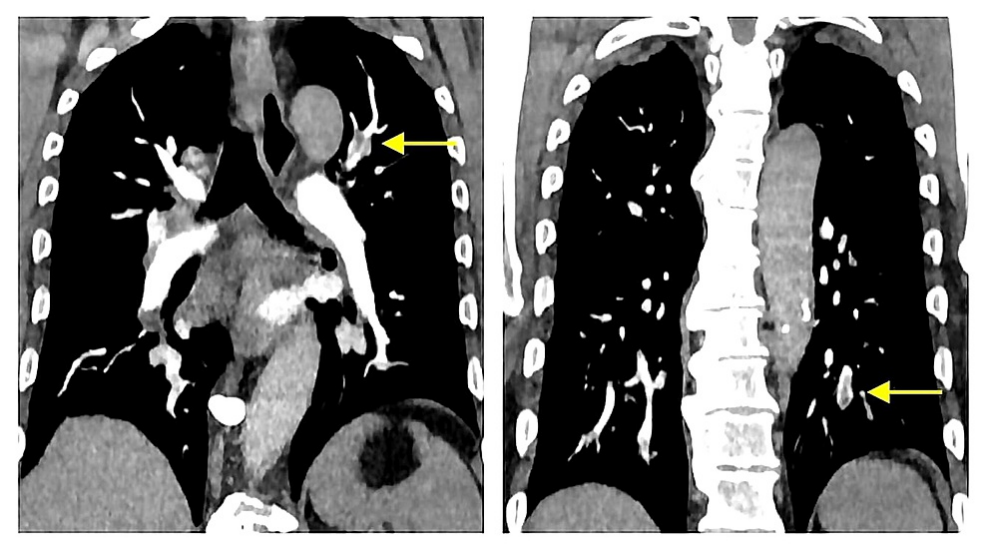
The computed tomography pulmonary angiogram showed a moderate burden of acute pulmonary emboli in branches to the left upper lobe, left lower lobe, and right lower lobe, and an embolus in the distal left main pulmonary artery
